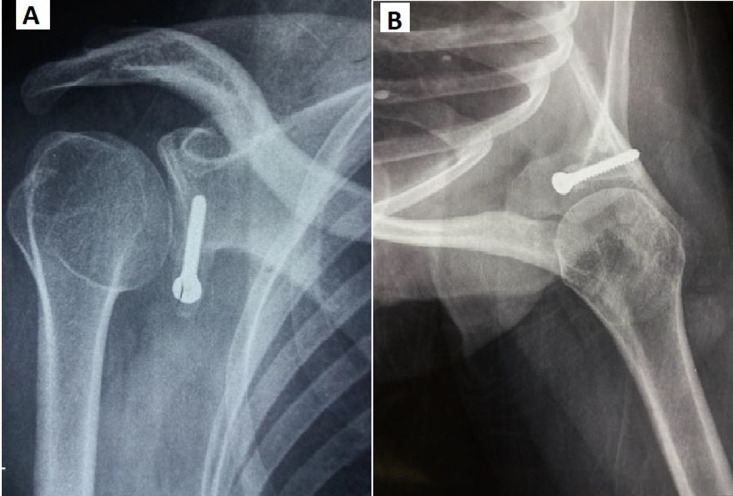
radiographie épaule post-opératoire; a) incidence de face rotation neutre; b) incidence profile de Bernageau
